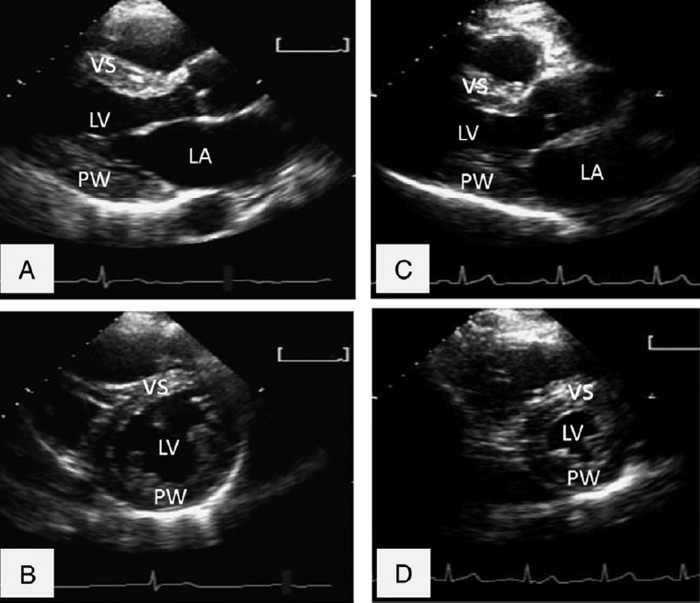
Athlete’s two dimensional echocardiographic images of left ventricle, (A) parasternal long axis view, (B) parasternal short axis view, comparison with healthy controls’ heart, (C) parasternal long axis view, (D) parasternal short axis view. VS:ventricular septum. LV:left ventricle. PW: posterior wall.
